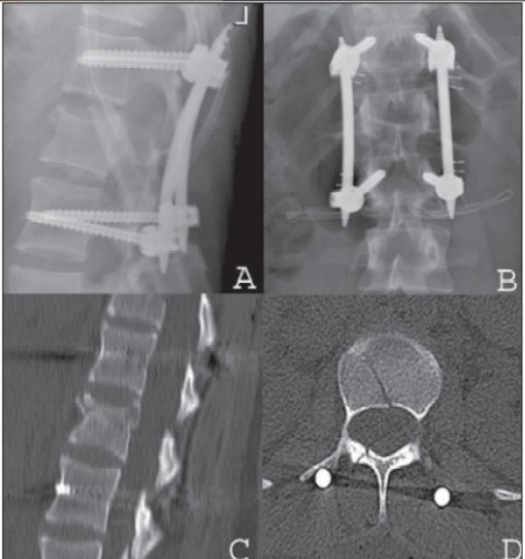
Post operative lateral (A) and AP X-ray (B), sagittal reconstruction CT (C) and axial CT (D) shows anatomic reduction and anterior height restoration. Uneventful recovery and percutaneous implant removal six months post trauma was performed
